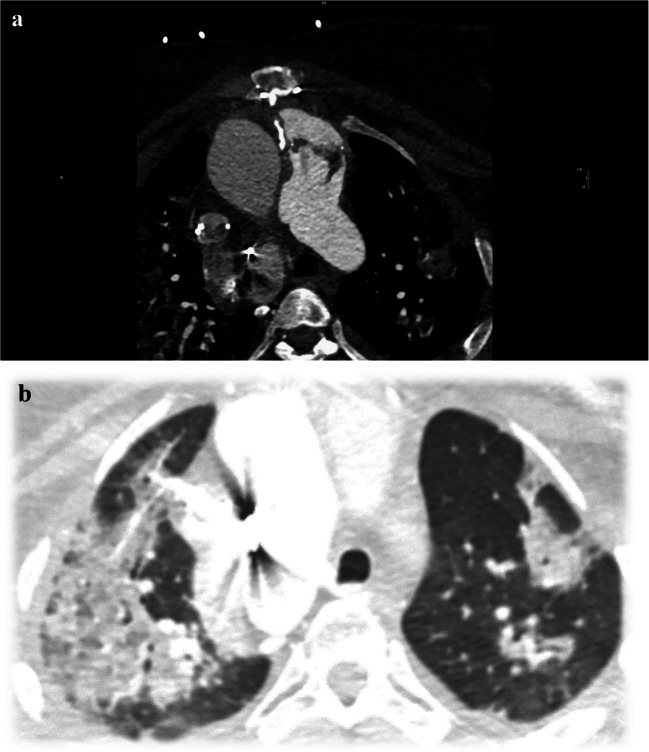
Adult patient s/p Rastelli procedure with prosthetic pulmonary valve. A) Cardiac CT video clip demonstrating prosthetic pulmonary valve with mobile vegetation. B) Chest CT with multiple septic pulmonary emboli. (Courtesy of Dr. Dan Wallihan.)
